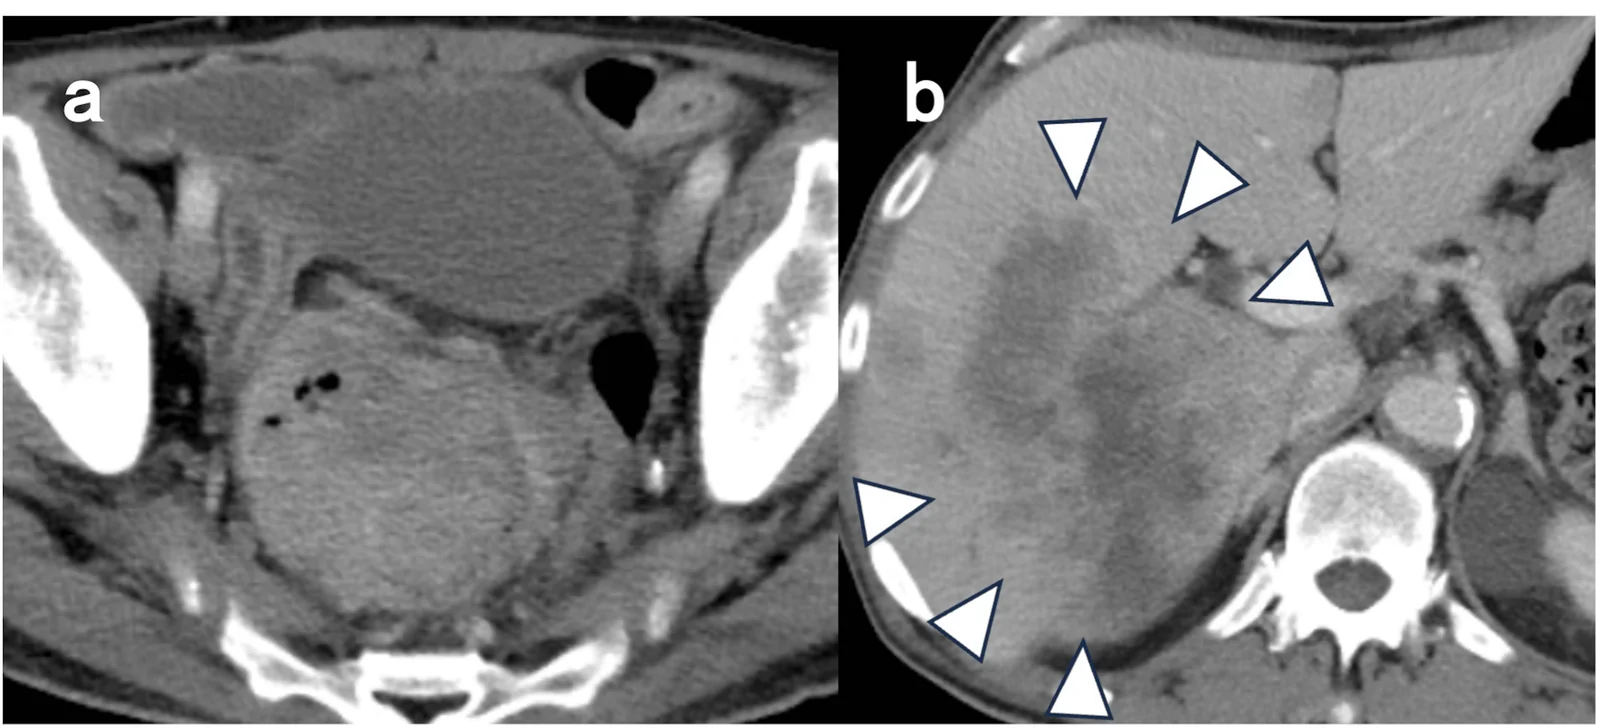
Contrast-enhanced computed tomography images before pembrolizumab treatment (a) Axial contrast-enhanced computed tomography image of the pelvis showing circumferential, contrast-enhanced wall thickening of the sigmoid colon. (b) Axial contrast-enhanced computed tomography image of the upper abdomen showing multiple hypoattenuating lesions in the right hepatic lobe. Arrowheads indicate the liver metastases.
